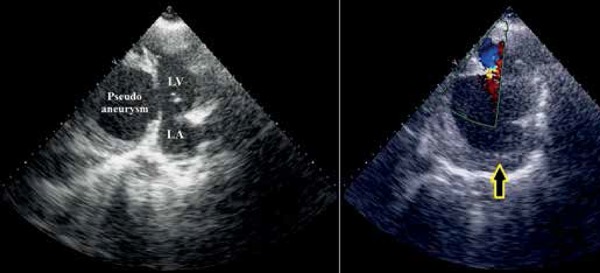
A, B: Transthoracic echocardiography showing large submitral
